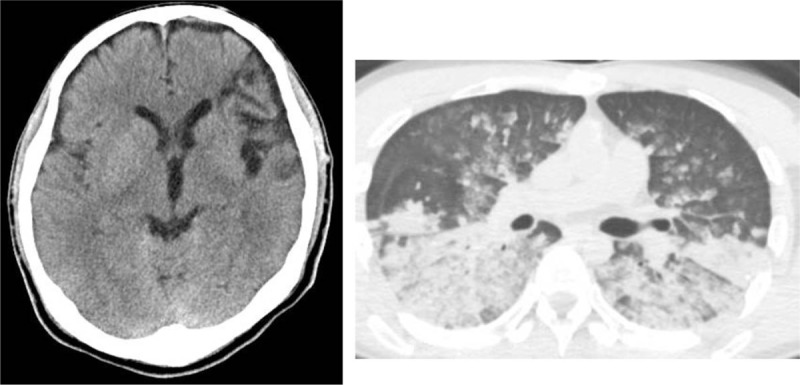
Head computed tomography (CT) showing no signs of hypoxic brain injury. Chest CT showed bilateral diffuse infiltrate suggesting cardiogenic pulmonary edema.
